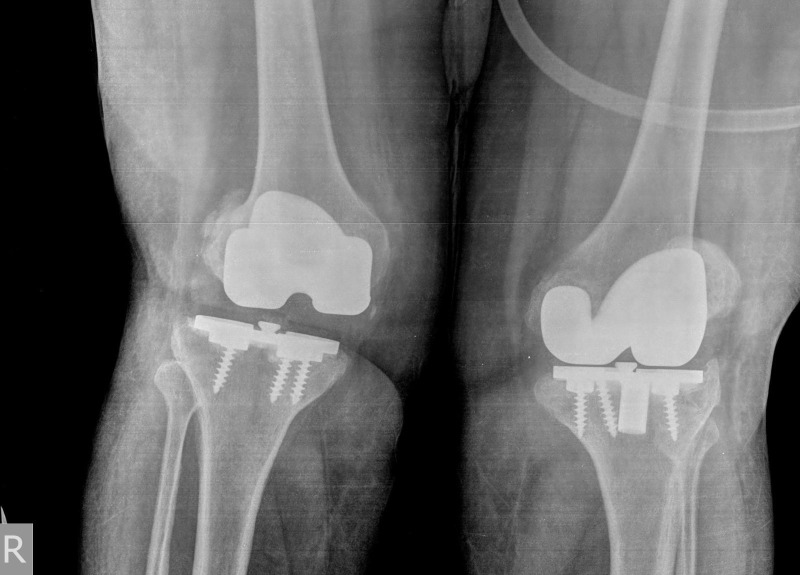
Preoperative anteroposterior (AP) standing radiograph showing bilateral failed total knee arthroplasties (TKAs).
